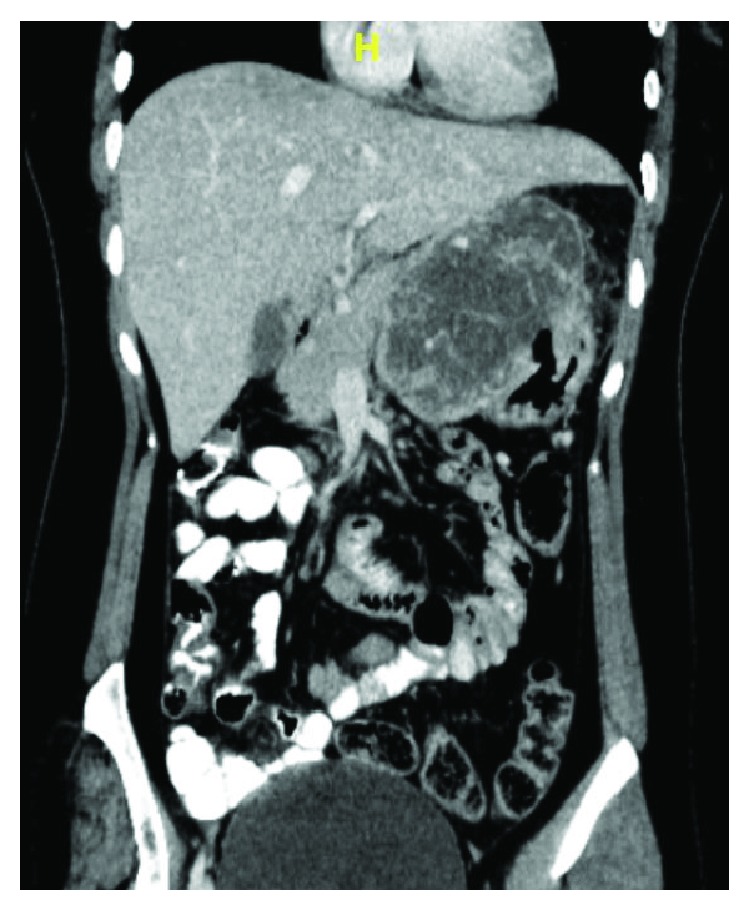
CT coronal section of the abdomen and pelvis showing a well-defined solid-cystic mass along lesser curvature of the stomach.
